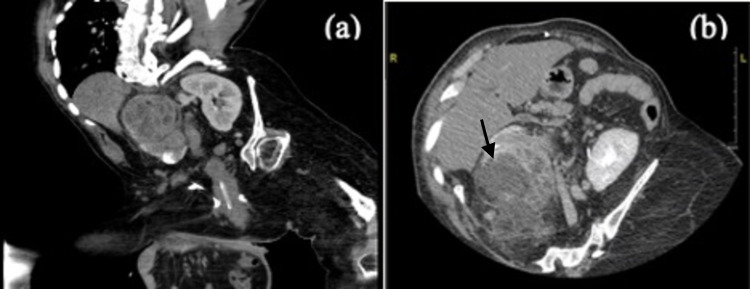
Patient’s abdominal CT scan (a) coronal view. (b) axial view. The images show a large mass in the upper lobe of the right kidney measuring about 9 x 8 x 6 cm, suggestive of xanthogranulomatous pyelonephritis CT: computed tomography
